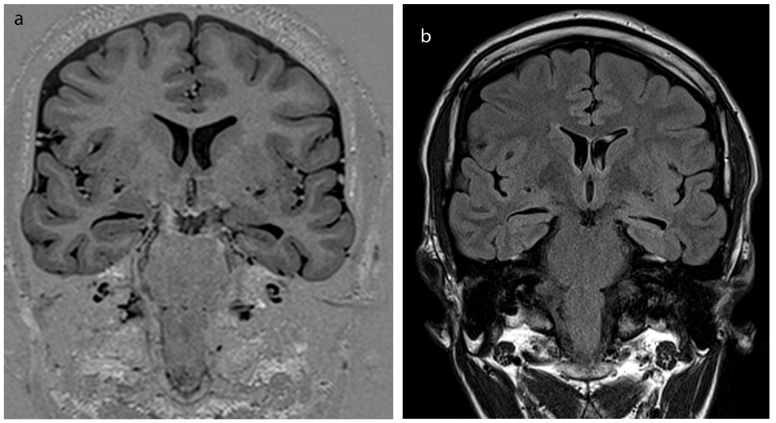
(A) High resolution epilepsy dedicated imaging of subject no. 19. Left hippocampal atrophy can be depicted from isotropic, coronal oblique T1-IR sequence. (B) FLAIR image also shows increased signal at the side of atrophy, revealing hippocampal sclerosis of the same subject.
